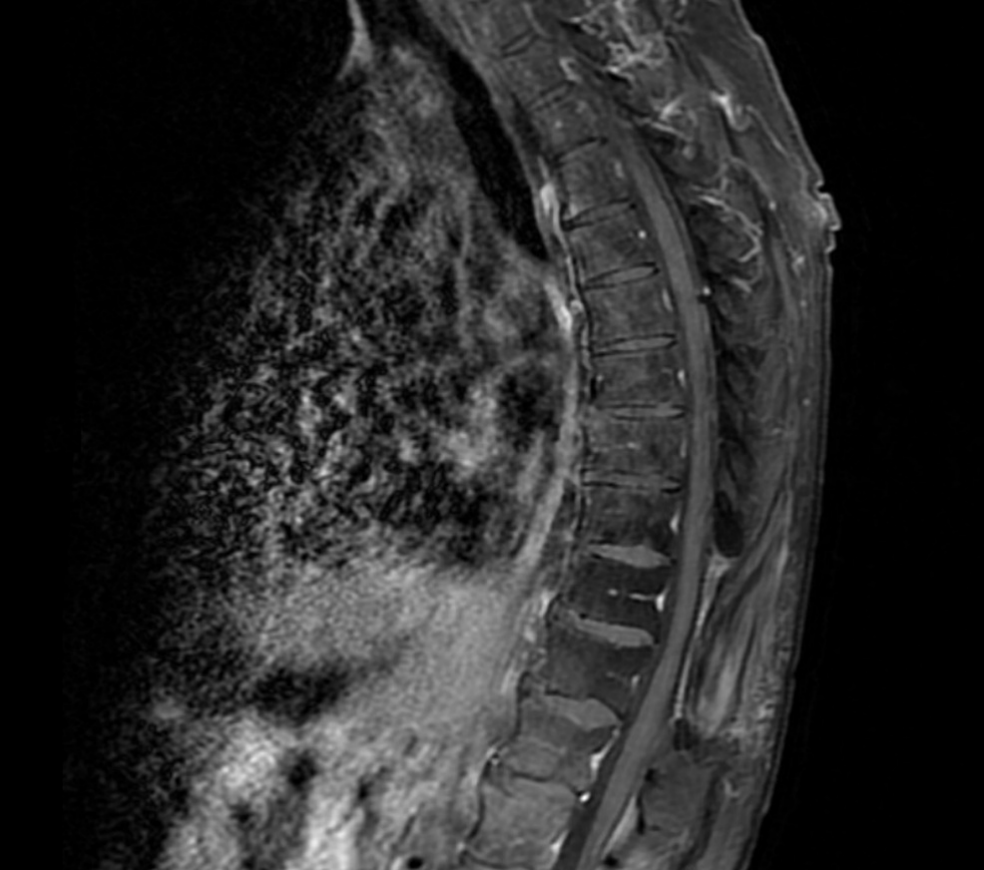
MRI of thoracic spine, T1 sagittal view with intravenous contrast and fat suppression No evidence of disease recurrence.
